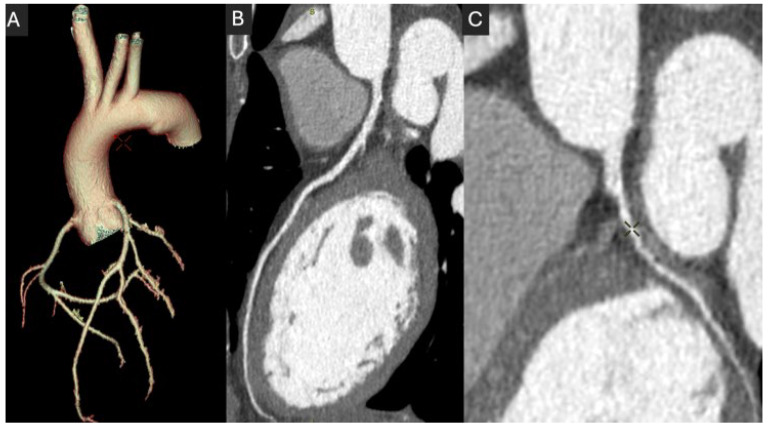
An example of a thoracic CTA in a 23-year-old woman with suspected Ehlers–Danlos syndrome, presenting with exercise-related chest pain. The scan was performed with a low-dose protocol (80 kV, 460 mA, and 0.23 s rotation), resulting in an effective dose of 1.13 mSv. The aorta and coronary arteries are visualized in a 3D volume-rendered reconstruction (A), with a full curved MPR at 90° rotation (B), and a zoomed-in curved MPR at 0° rotation (C), focusing on the left anterior descending artery (LAD). The image quality was optimal, allowing us to rule out both coronary and aortic disease.
